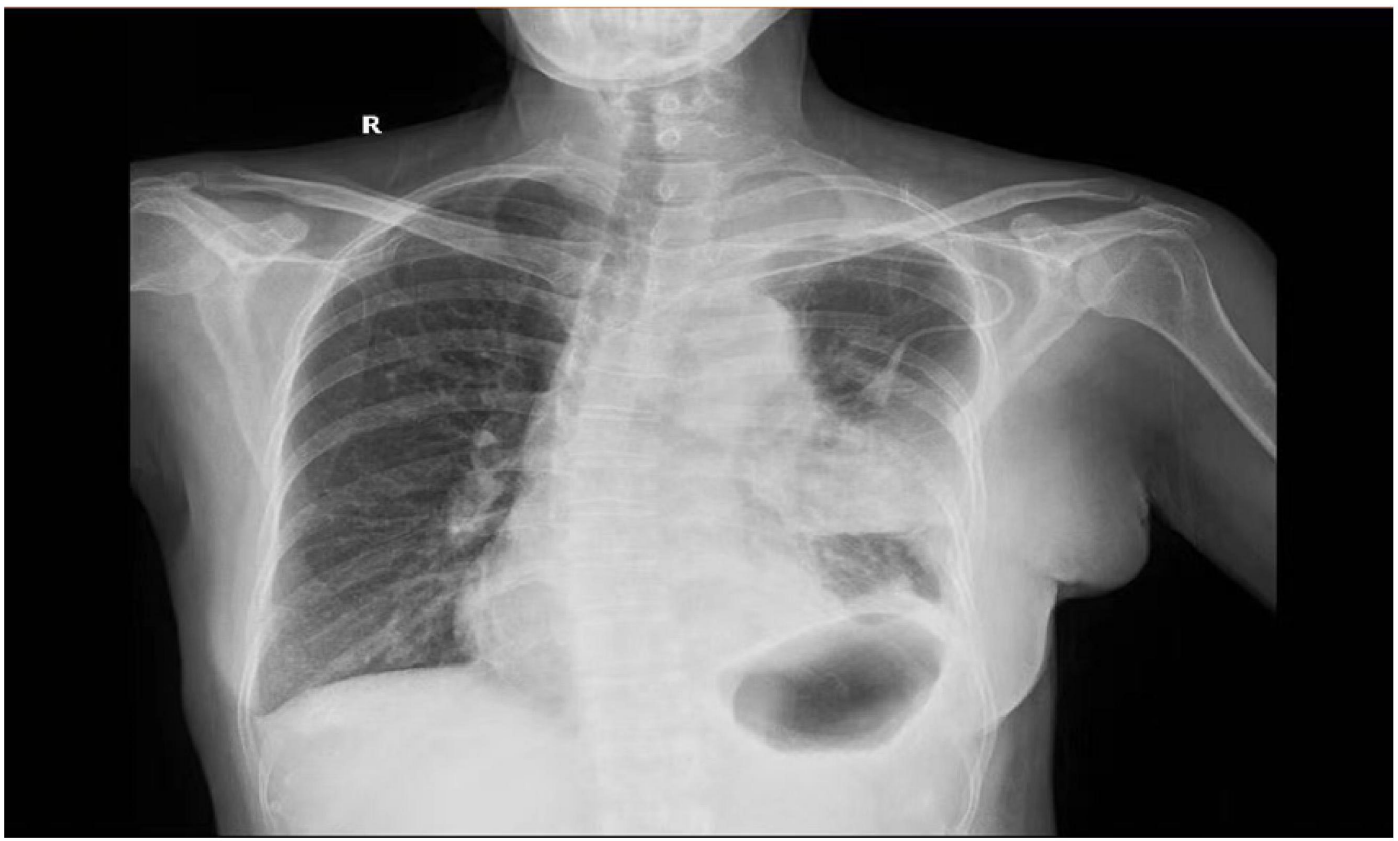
Chest X-ray of the patient half month after the operation. (2023.4.14).
